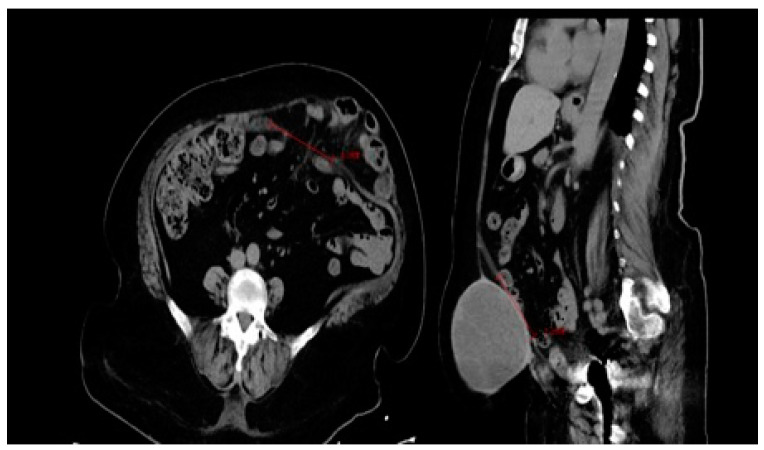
CT image revealing recurrence and the presence of a granuloma.
